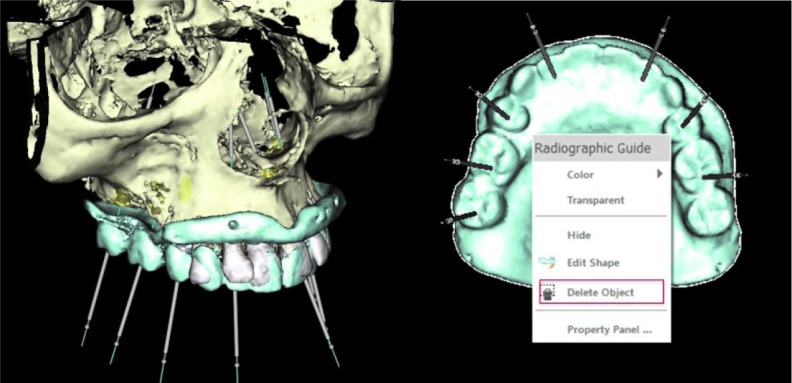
a: Implants planned in the planning software by merging the patient scan with the scan of the modified radiographic template, b: Mod-ified radiographic template deleted following implant planning
